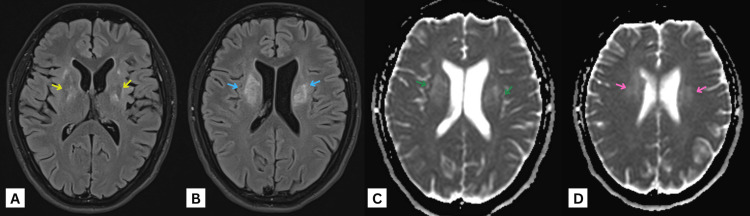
MRI of the brain axial FLAIR images at the level of the (A) basal ganglia and (B) corona radiata, and MRI of the brain axial ADC images at the level of the (C) corona radiata and (D) parietal cortex, showing bilateral, almost symmetrical, signal alteration in the form of T2-FLAIR high-signal intensity involving the globus pallidus (yellow arrows), corticospinal tracts, and corona radiata (blue, green, and pink arrows). It starts from the corona radiata superiorly at the level of lateral ventricles extending to cerebral peduncles of the midbrain (blue arrows) inferiorly, affecting the right side more at this level. Similar signal alteration is also noted at the right anterolateral side of the pons. No diffusion restriction can be seen. MRI: magnetic resonance imaging, FLAIR: fluid-attenuated inversion recovery, ADC: apparent diffusion coefficient
